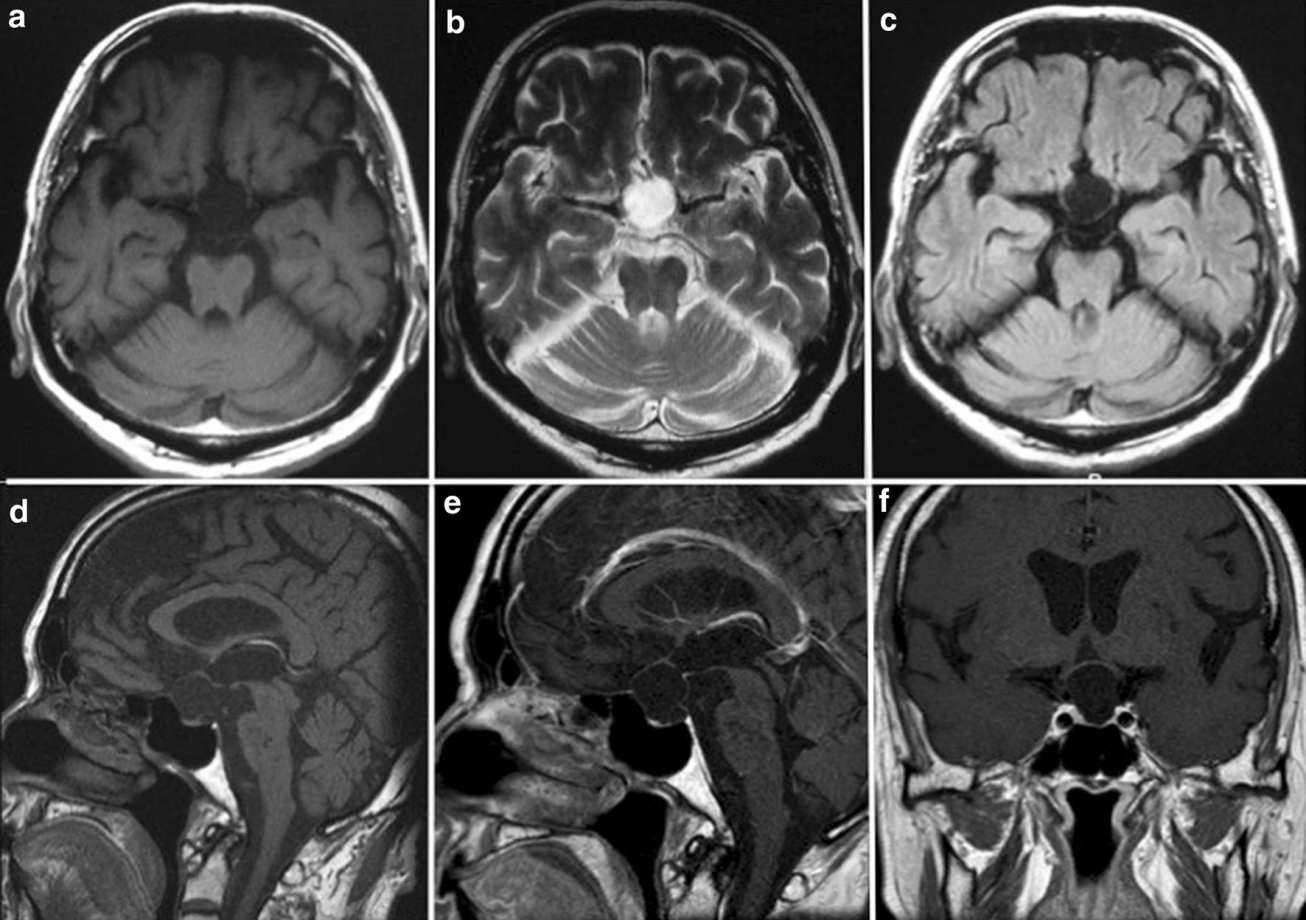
Preoperative MR imaging showing a cystic mass in the sellar region. The cystic contents displayed low intensity on T1-weighted MR imaging, high intensity on T2-weighted MR imaging, and low intensity on FLAIR imaging. No enhancement was evident after contrast medium administration (a axial T1-weighted MR imaging; b axial T2-weighted MR imaging; c axial FLAIR imaging; d sagittal T1-weighted MR imaging; e sagittal gadolinium-enhanced T1-weighted MR imaging; f coronal gadolinium-enhanced T1-weighted MR imaging)
